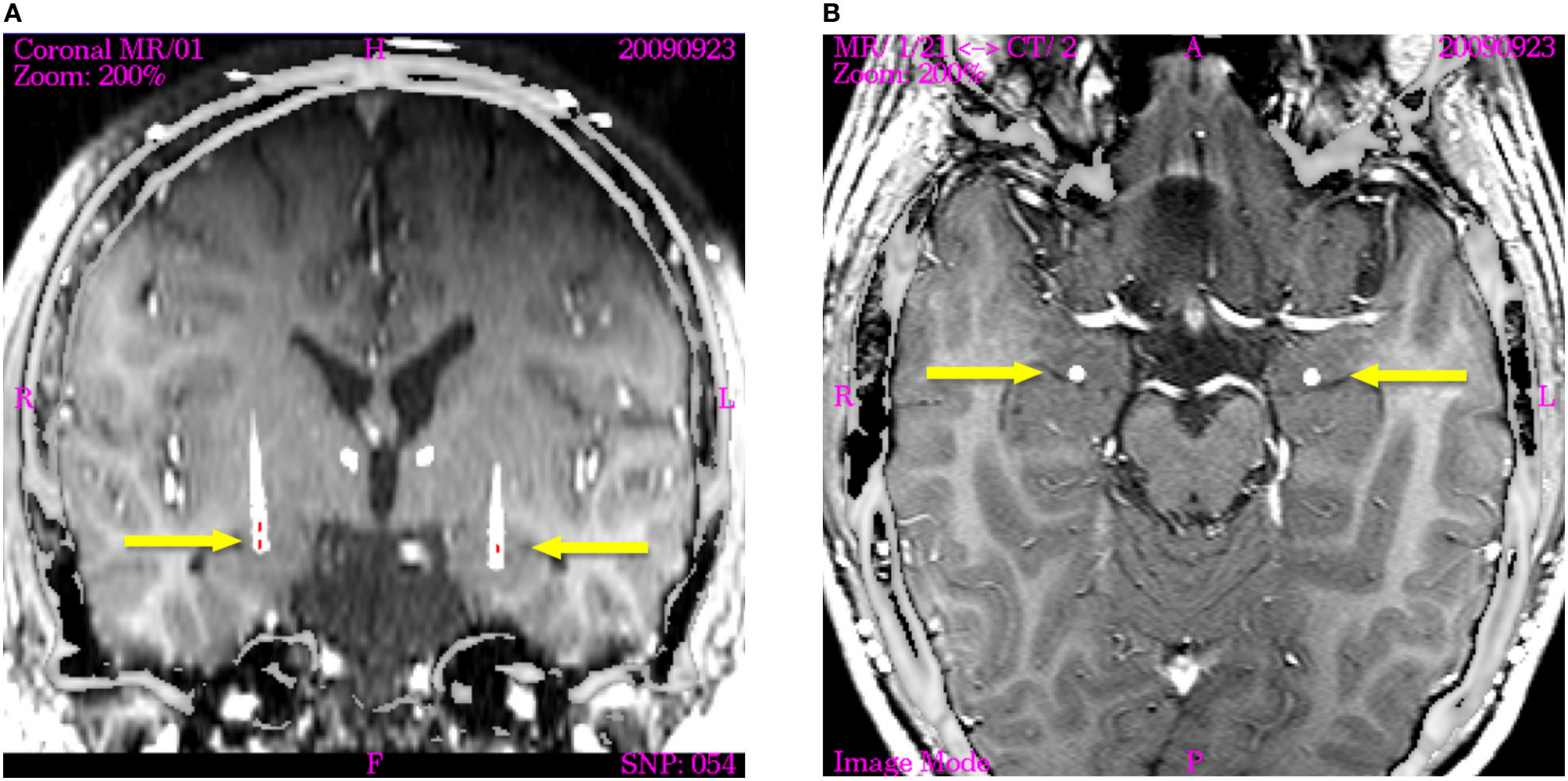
Image fusion of post-operation CT (nine months after implantation) with T_1_-weighted intraoperative planning MRT visualizing the actual electrode position in axial and coronal planes (displayed image size: 145 × 145 mm). The active contacts are indicated by red lines in the coronal section. (A) The coronal image is re-sliced in order to show the electrode trajectory in the section plane. Arrowheads indicate the trajectories of the supra-amygdaloid electrodes, which are not activated. The active poles on the right electrode are located close to the posterior part of the basolateral amygdaloid nucleus (Contact 0 and 1). The active pole on the left electrode is positioned in the center of the basolateral nucleus. (B) Horizontal section through the amygdala of both hemispheres showing the electrodes indicated by arrows in the posterior part of the basolateral nucleus immediately in front of the tip of the temporal horn of the ventricle.
